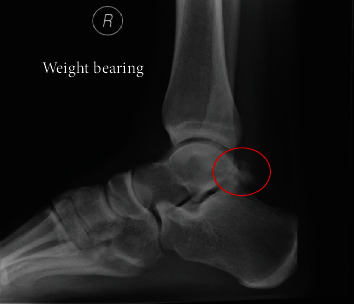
Lateral radiograph of right ankle showing an os trigonum and posterior talus process fracture.
